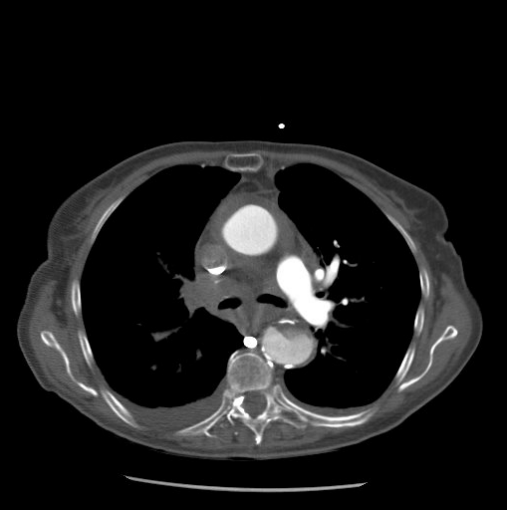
CT image showing compression of the right pulmonary artery following repair of the aortic dissection. This image demonstrates the aortic graft (G) and the peri-aortic haematoma still compressing the right pulmonary artery (RPA).
